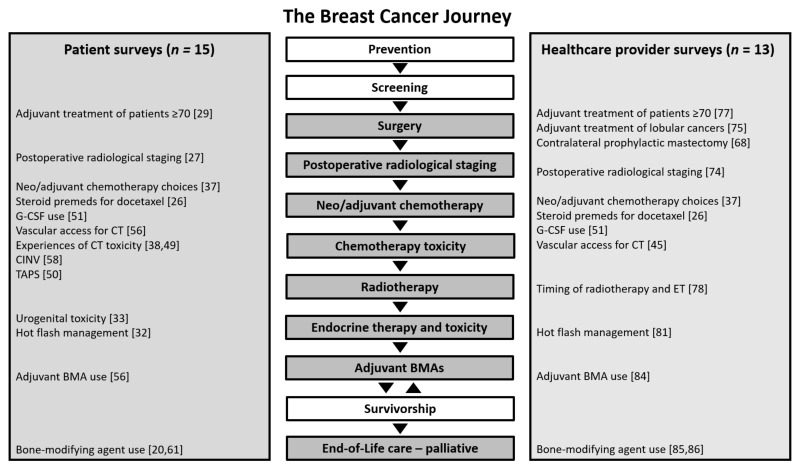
The breast cancer journey: where our surveys fit and where gaps exist. BMA = bone-modifying agent, CINV = chemotherapy-induced nausea and vomiting, CT = chemotherapy, ET = endocrine therapy, G-CSF = granulocyte colony stimulating factor, TAPS = taxane-associated pain syndrome.
